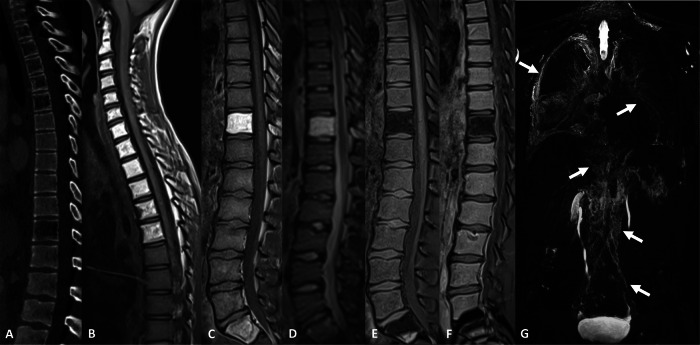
Bone marrow heterogeneity discovered on chest CT performed for recurring pulmonary infections in an 11-year-old male, revealing primary diffuse lymphangiectasis. A Noncontrast CT, sagittal view. Areas of osteolysis with fat attenuation density involving multiple vertebral bodies, without cortical destruction. B, C T1w, sagittal view. Corresponding anomalies demonstrating high signal intensity on a T1w image, corresponding to intralesional fat. D T2w, sagittal view. Anomalies also exhibit high signal intensity on T2w images. E, F T1w DIXON, water, before and after contrast administration, respectively, sagittal view. Absence of intralesional enhancement. Differentials include vertebral body hemangioma. G 3D FRFSE T2w, MIP reconstruction (20 mm), coronal view. Reveals supra- and infradiaphragmatic lymphangiectasis (arrows), including subpleural, intrapulmonary, retroperitoneal, and pelvic lymphangiectasis. The associated diffuse lymphangiectasis orients the vertebral anomalies to be of lymphatic origin
